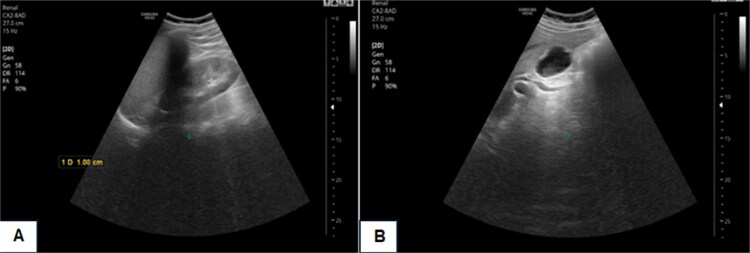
Abdominal ultrasound showing a mild liver fatty infiltration. The gallbladder was normal in size with a small sludge (white arrow in image B) measuring 0.8 × 0.4 cm.
